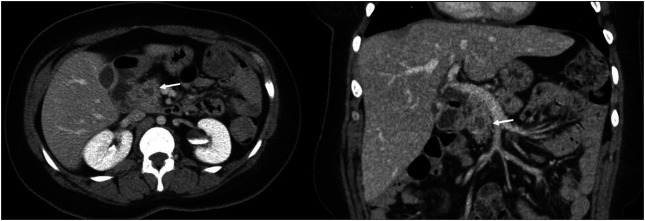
Computed tomography showing a stable 10 mm hypoenhancing mass of the inferior pancreatic head with persistent pancreatic ductal dilation (6 mm) after 9 months of amoxicillin therapy.
